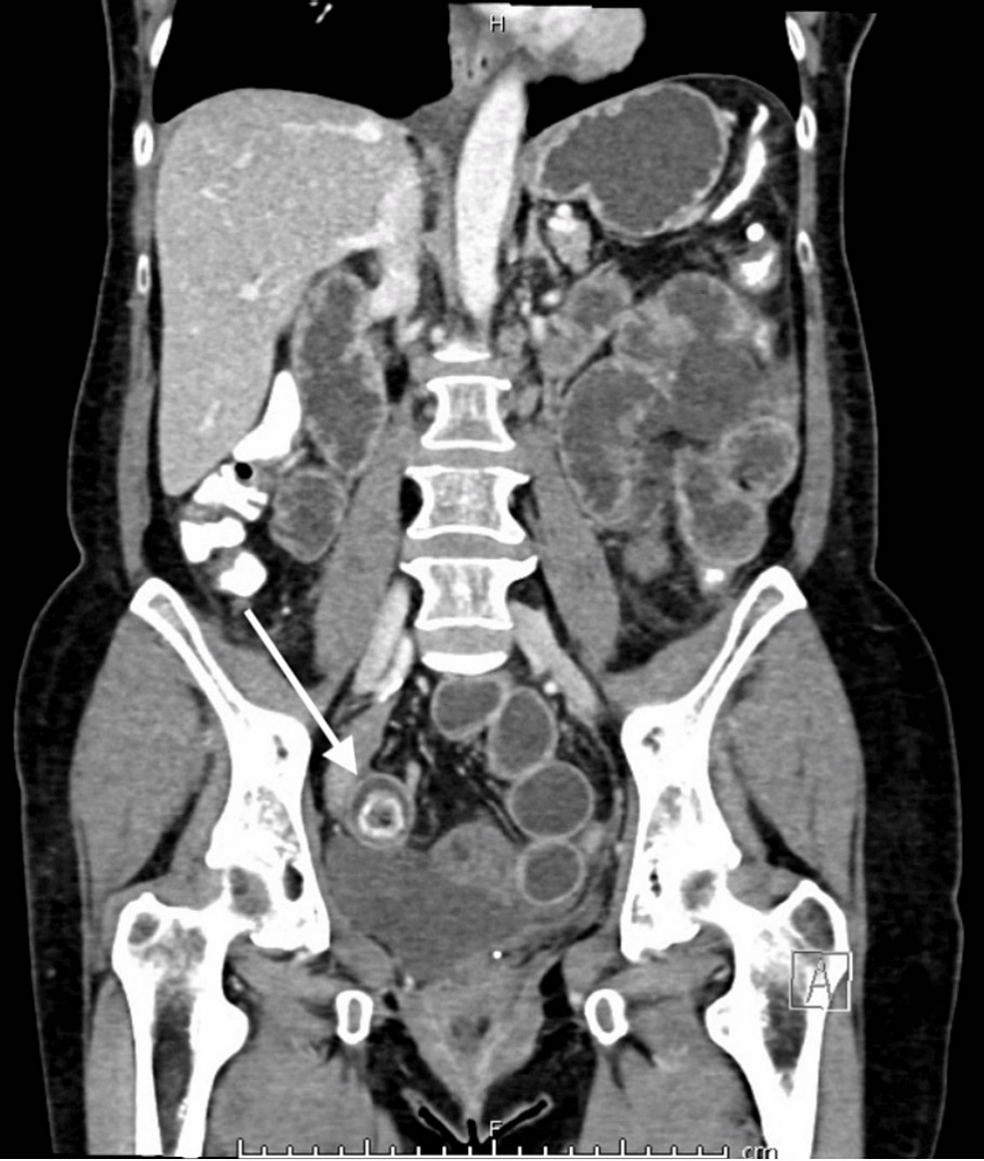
Repeat CT AP during the patient’s second admission CT AP: Computed tomography scan of the abdomen and pelvis This coronal view of CT AP demonstrates high-grade small bowel obstruction secondary to an enterolith (white arrow), which has migrated to the distal ileum from a jejunal diverticulum.
